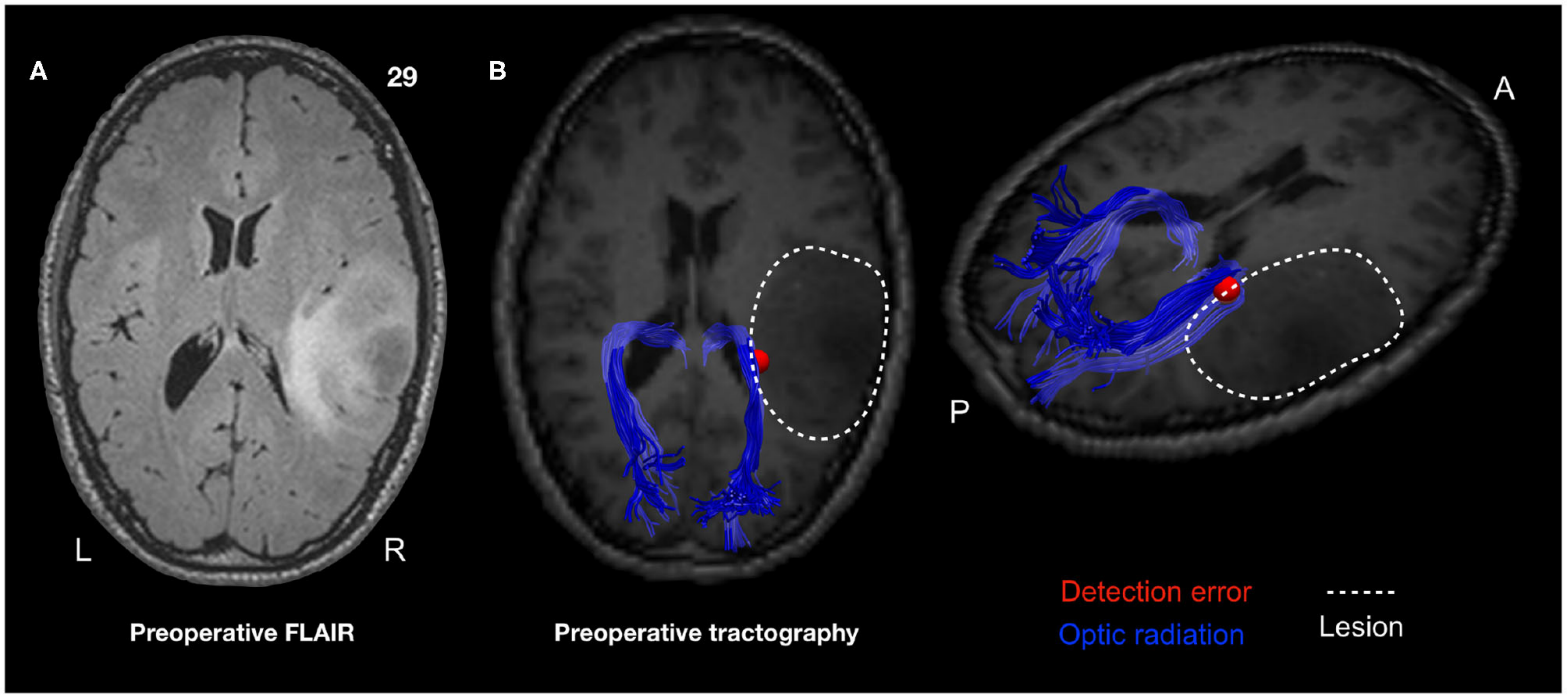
A case of presumptive right fronto-parietal lower-grade glioma in a 32-year-old female, operated on in asleep-awake-asleep anesthesia with the aid of motor, cognitive, haptic, language and visual (iVT) mapping. The axial FLAIR is in the upper left panel (A). DES (LF 3 mA) interfered in multiple sites during iVT performance. The location of one of these (giving detection errors) is reported as a red dot in the pre-operative postGd T1 weighted MR to which the reconstructed OR (in blue, HARDI) are coregistered and superimposed (B). The location of the site is within the lateral border of OR, in an area of tumor infiltration; the tumor volume is indicated with a dotted line (middle and right upper panels show the axial and the lateral view, A, anterior; P, posterior; L, left; R, right). Resection was stopped at this level, resulting in a subtotal resection. The histo-molecular diagnosis revealed grade II IDH1 wt astrocytoma.
